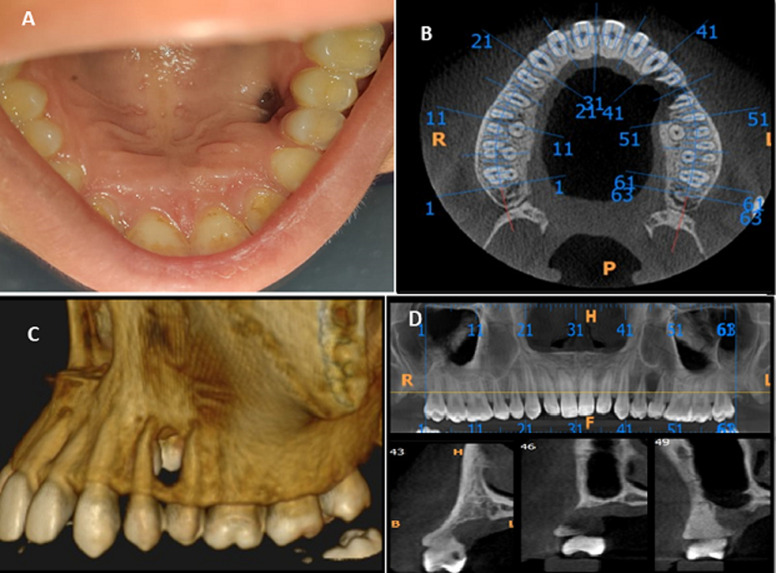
A) intraoral photograph showing palatal perforation in the maxillary left premolar region; B) axial section of cone beam computed tomography (CBCT) showing bony destruction between maxillary left premolar region; C) 3D reconstruction image showing through and through perforation in left maxillary premolar region; D) aggressive destruction of buccal and lingual cortical plate with perforation in floor of maxillary sinus in left premolar region was noted with external root resorption
